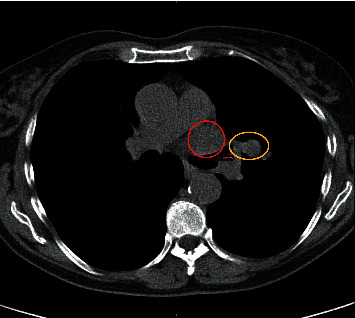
Screening chest CT scan that originally identified the 19 × 22 mm nodule in the left upper lobe of the lung (red circle). Left hilar adenopathy was also noted on presentation (yellow circle). Intralobular septal thickening was also noted.
